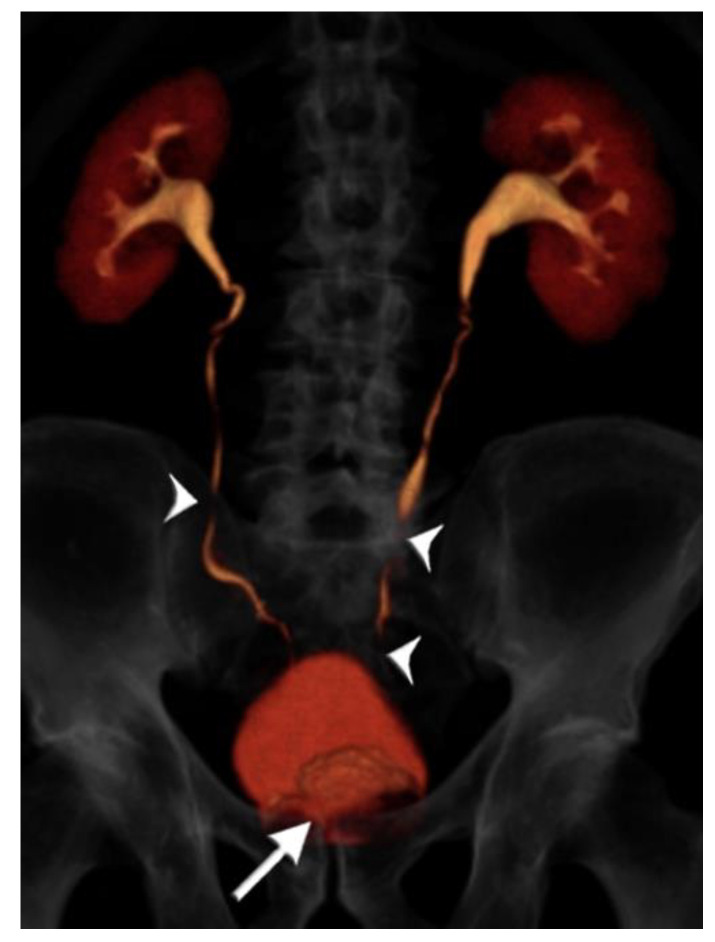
75 year-old male with gross hematuria imaged with single-bolus CT urography. Volume-rendered image of the excretory phase shows near complete contrast opacification of the ureters, apart from very short unopacified segments (arrowheads). There is an enlarged prostate indenting the urinary bladder base (arrow). There is no upstream hydroureteronephrosis and the image quality was deemed adequate. The total table time was 11 min and the dose-length product was 870 mGy·cm.
